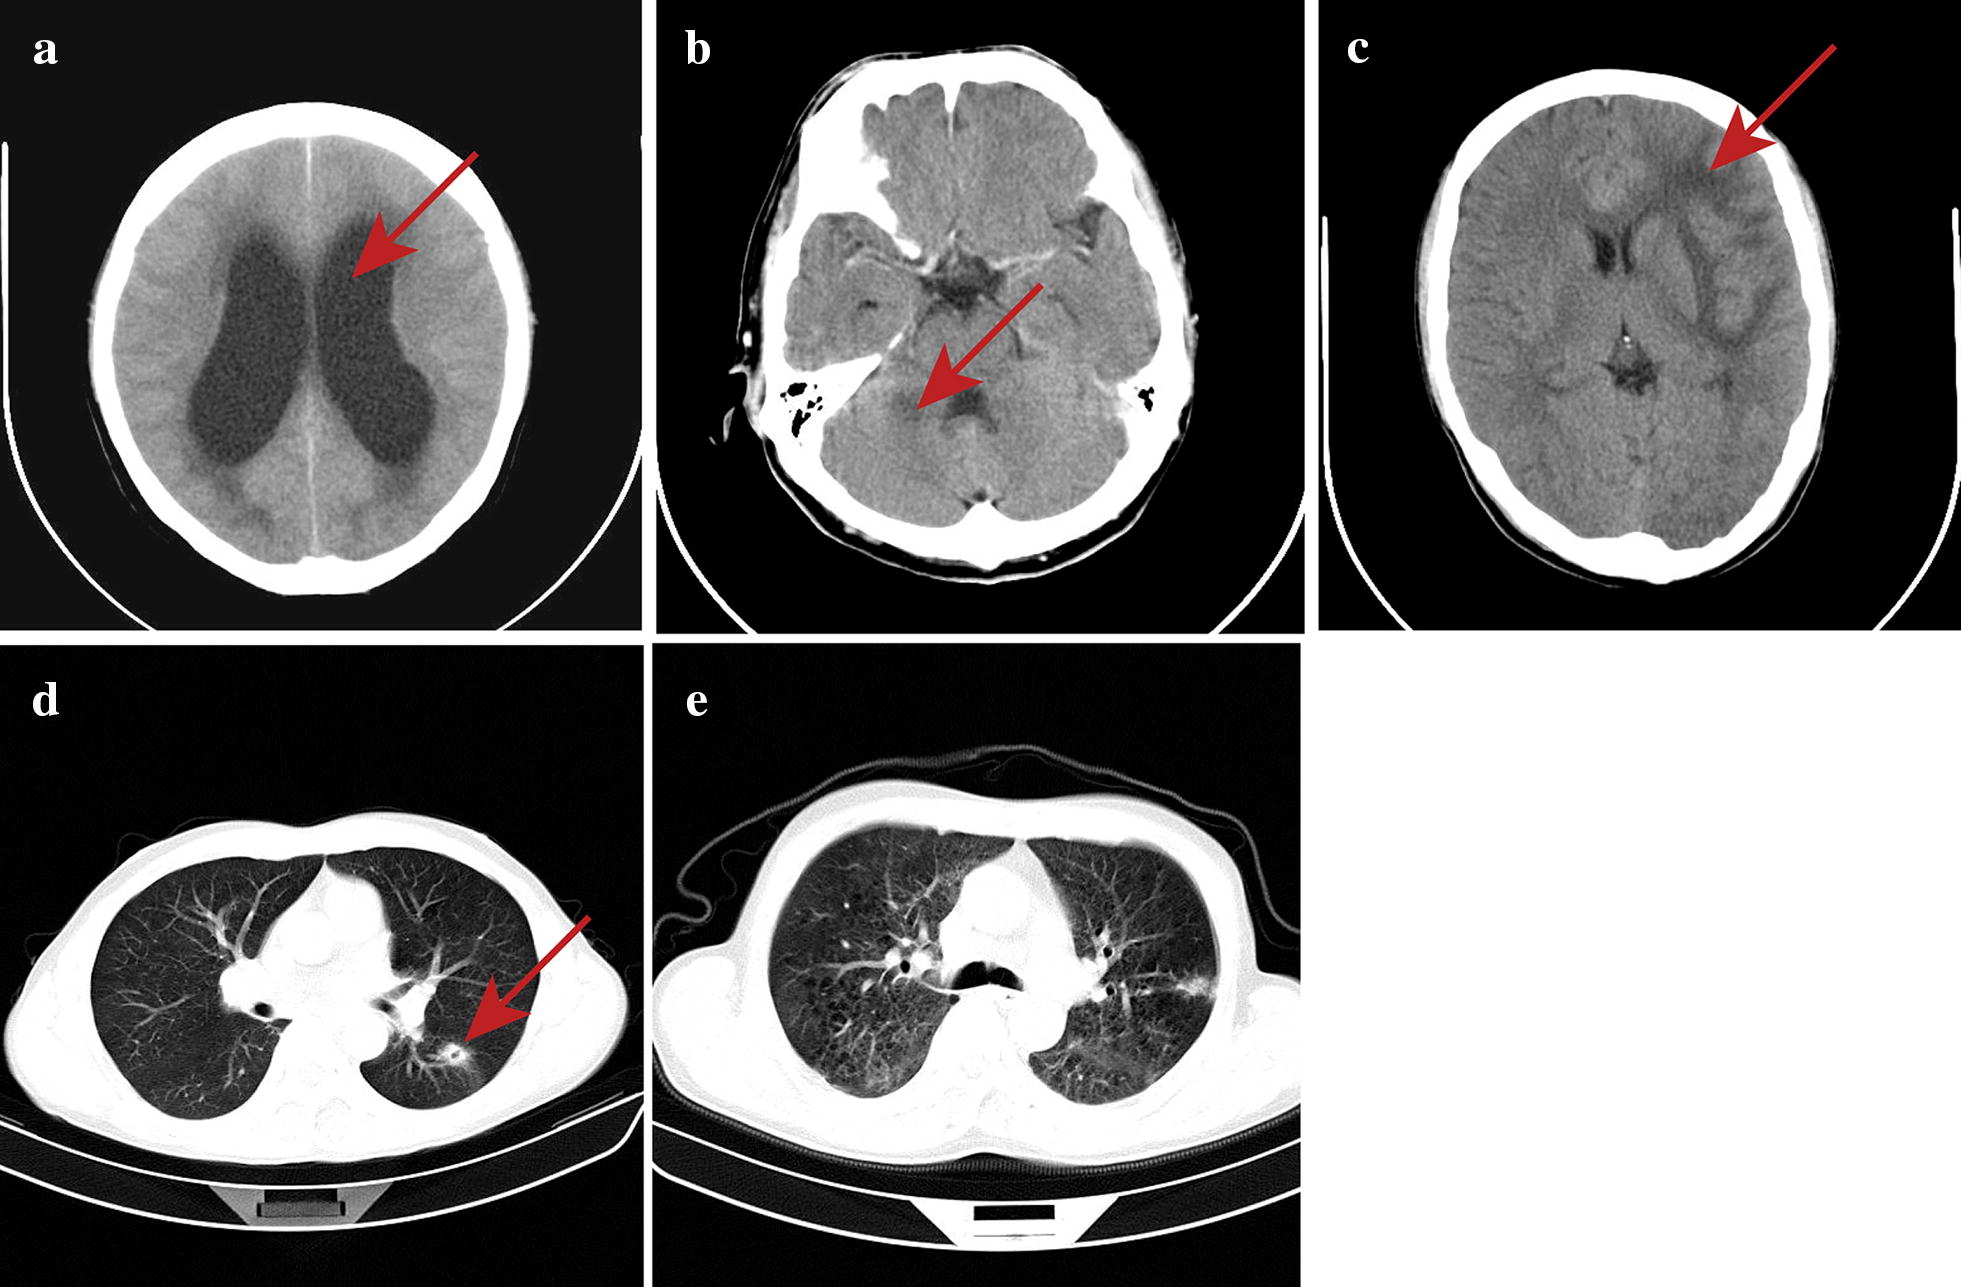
Brain and lung CT examination of the patients with AIDS-associated *T. marneffei* central nervous system infection. a Bilateral lateral ventriculomegaly: the bilateral lateral ventricles were dilated, and the brain parenchyma was slightly compressed bilaterally (arrow). b Patchy low-density shadow in the right cerebellar hemisphere in contrast enhanced CT: flaky low-density shadow on the right cerebellar hemisphere with unclear boundaries; the lesion was approximately 2 cm in size. There was no enhancement of the lesions after the use of contrast media (arrow). c Multiple intracranial infectious lesions with cerebral edema: parenchymal swelling in the left frontal lobe, insula and basal ganglia, cerebral sulcus thinning, extensive edema in the white matter parenchyma of the frontal lobe, showing low density (arrow). d Left pulmonary nodules with voids: high-density nodules in the dorsal segment of the left lower lobe, approximately 2 cm in diameter, with unclear boundaries and a cavity within the lesion (arrow). e Interstitial pneumonia: diffuse ground-glass opacity of bilateral lungs, patchy high-density shadow of the subpleural area in the posterior segment of apex of left lung, with thickening of the adjacent pleura
